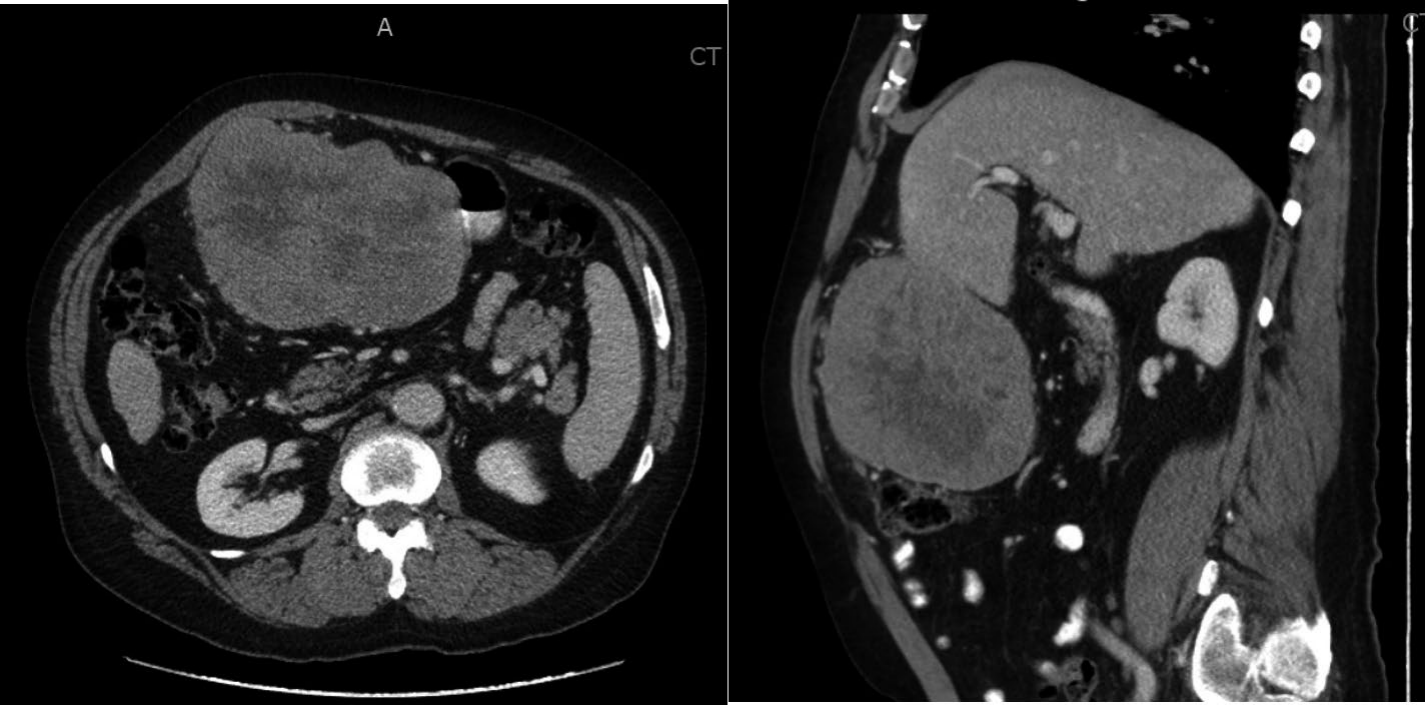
CT scan of the large, pendular mass originating from the left lobe of the liver. Note the hypodense area inside the neoplasm suggestive of necrosis. The left image shows the stomach intimately associated with the mass with transverse colon sitting posteriorly. The image on the right shows the mass compressing the anterior abdominal wall. He is staged as a T3N0M0
